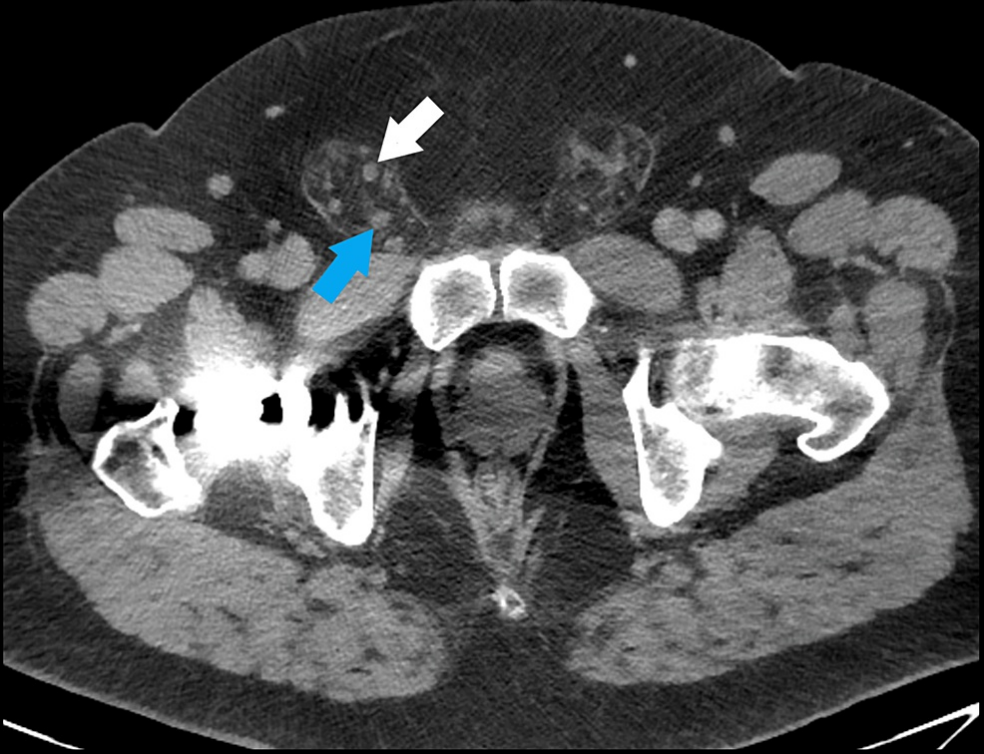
Pre-operative CT showing proximal right ureter (white arrow) and distal right ureter (blue arrow) within a right inguinal hernia.
